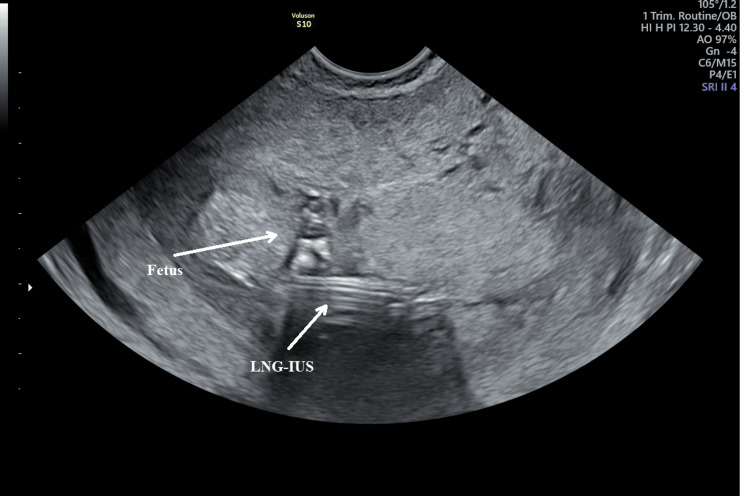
Ultrasound image demonstrating a viable intrauterine pregnancy at 12 weeks’ gestation with the LNG-IUS located in the uterine fundus. LNG-IUS: levonorgestrel-releasing intrauterine system.
